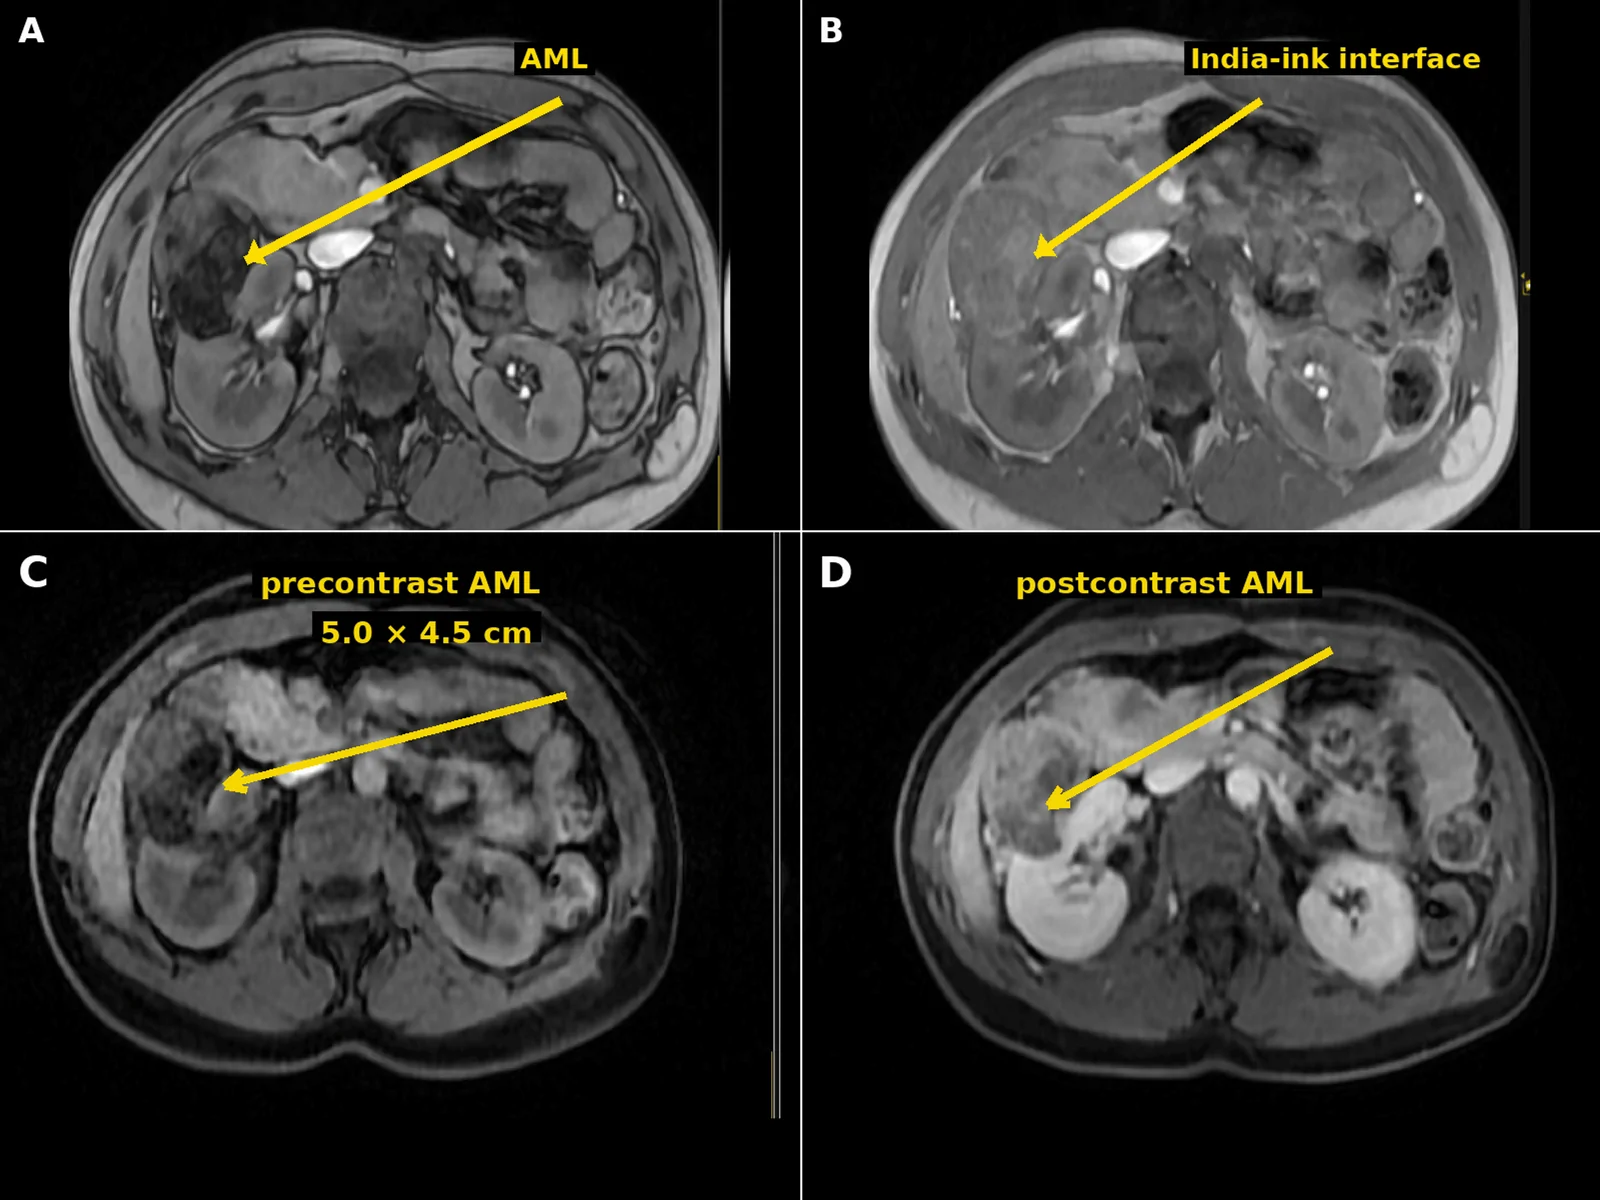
Preprocedural MRI The in-phase image identifies the right renal angiomyolipoma (A). The opposed-phase image demonstrates the characteristic India-ink artifact at the fat-water interface (B). On the displayed axial image, the lesion measures 5.0×4.5 cm. Precontrast (C) and postcontrast (D) fat-suppressed T1-weighted images show the intralesional macroscopic fat and enhancing soft-tissue component. Yellow arrows identify the lesion and the relevant imaging features. AML: angiomyolipoma; MRI: magnetic resonance imaging
